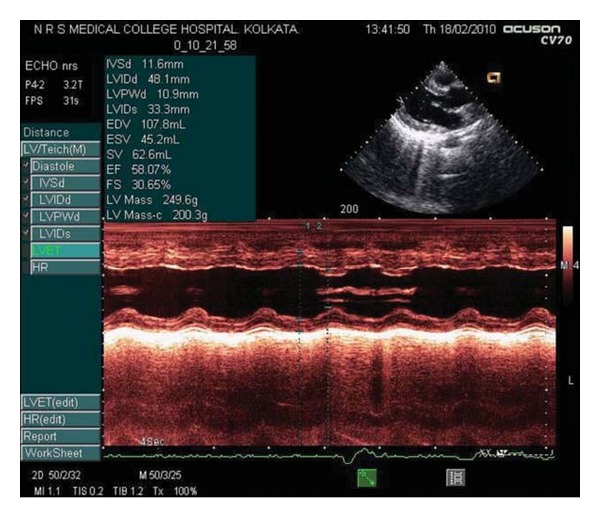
Posttreatment echocardiography showing marked improvement of left ventricular systolic function.
